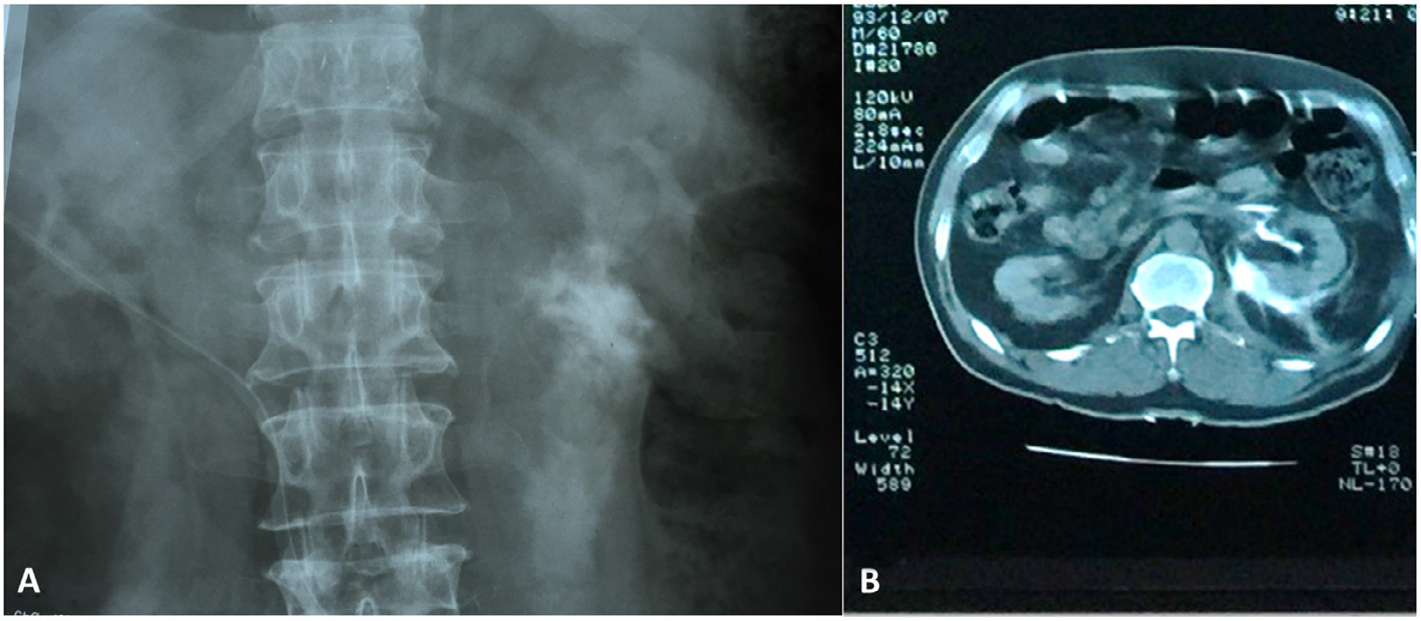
Intravenous pyelogram (A) and abdominal computed tomography (B) show fluid extravasation into the retroperitoneal space at the left side.
